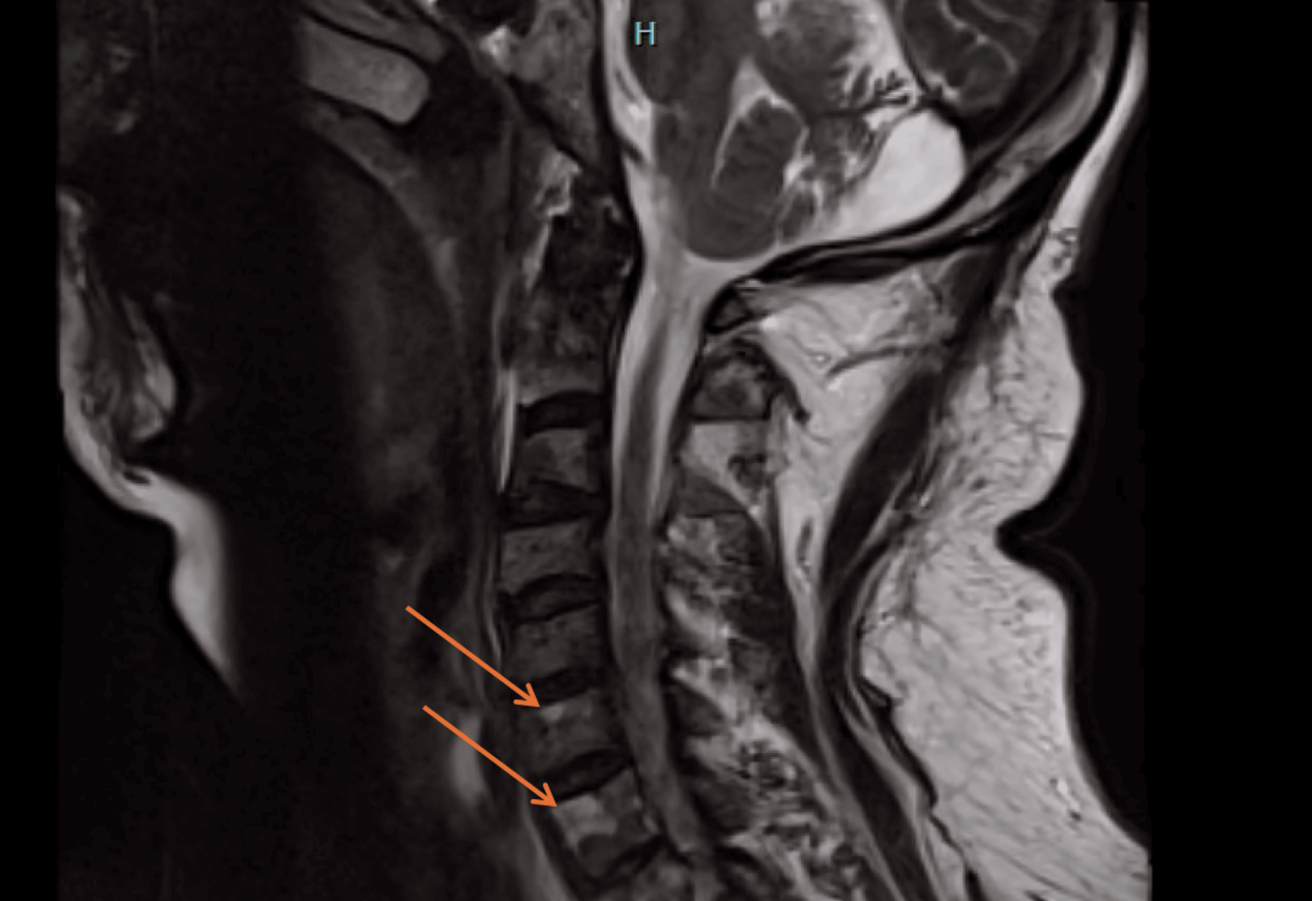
T1 sagittal magnetic resonance image of the cervical and thoracic spine. Multiple enhancing hypointense lesions throughout the cervical spine (orange arrows).
